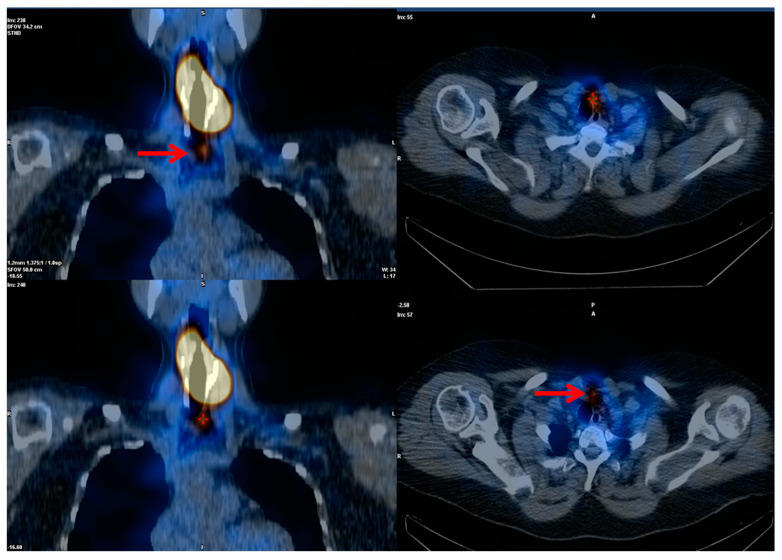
Post-treatment high-activity ^131^I whole-body scintigraphy. Large thyroid remnant with a small single iodine-avid lymph node metastasis (red arrows) at central neck compartment.
